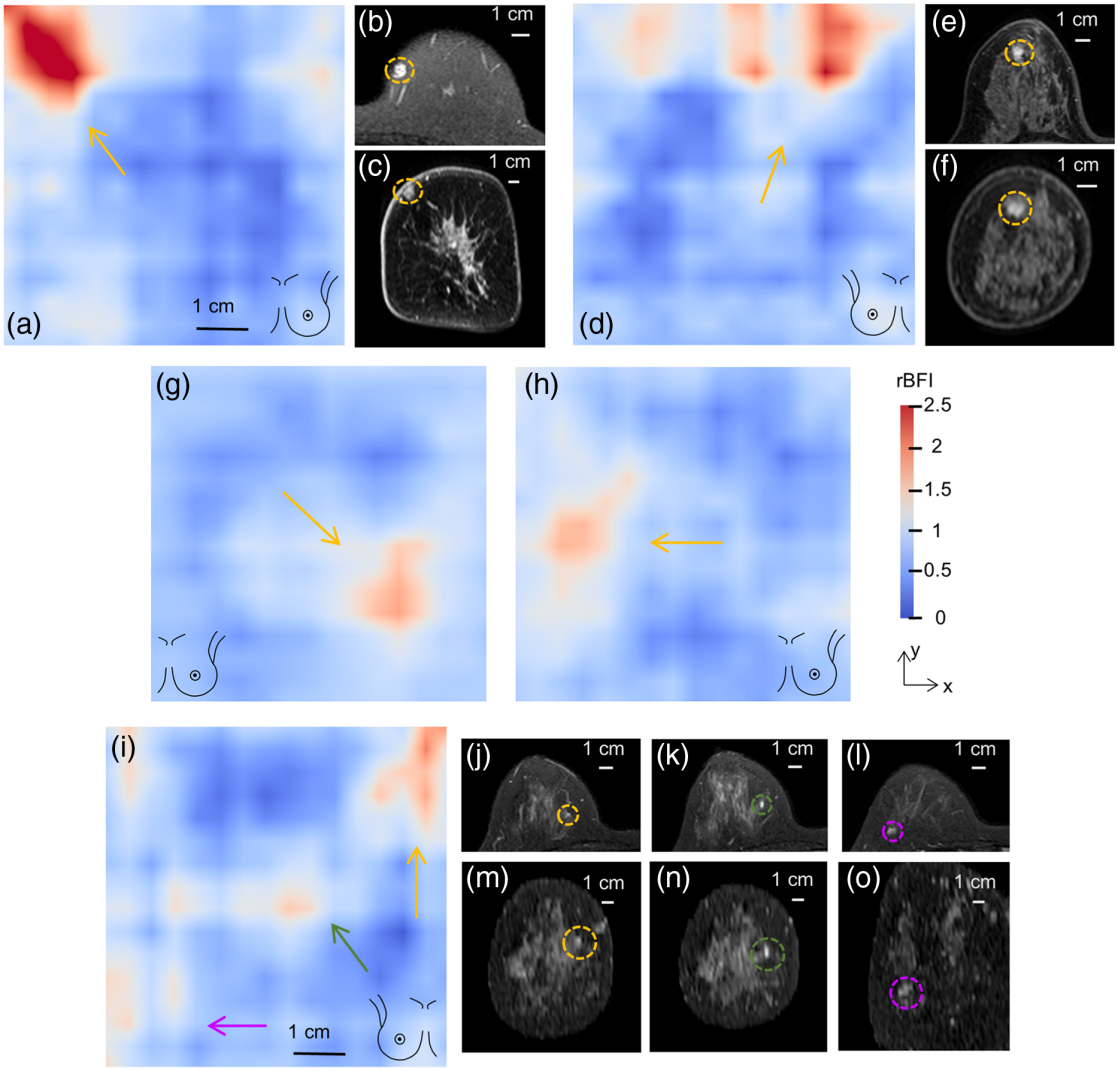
Representative DCT and MRI images that contain lesions. (a) A malignant lesion appeared on the upper left quadrant and corresponded to the presence of a nodular long T2 signal shadow observed in the MRI images with (b) foot-to-head orientation and (c) anterior-to-posterior orientation, also located within the upper left quadrant of the breast. The higher rBFI exhibited a larger extent of the region when compared with the region of nodular long T2 signal shadow (measuring 0.80  cm×0.84  cm in size). (d) A malignant lesion indicating small differences between DCT and MRI images. The nodular high T2 signal shadow was observed in the MRI images and appeared within the (e), (f) upper left quadrant (about 11 o’clock, measuring 0.87  cm×0.98  cm in size) of the right breast, whereas the high blood region was observed on the upper right quadrant. (g) A benign lesion (cyst) appeared around 3 to 4 o’clock and corresponded to the high blood region of the left breast. (h) A benign lesion (fibroadenoma) appeared on the upper quadrant, whereas the high blood region was observed on the left quadrant. (i) Three benign lesions were located in the upper right, middle, and lower left, respectively. These regions closely corresponded to the presence of three nodular high T2 signals observed in the MRI images and appeared within the (j), (m) inner upper quadrant, (k), (n) inner quadrant, and (l), (o) outer lower quadrant of the right breast, respectively. The high rBFI also exhibited a larger extent of region when compared with the region of nodular high T2 signals, with the largest lesion being measured as 0.69  cm×0.56  cm.
